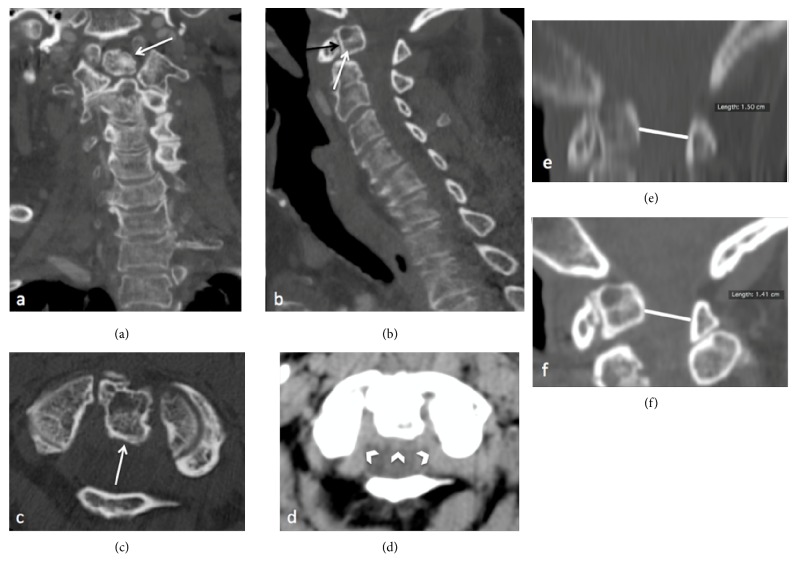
Angiography brain CT obtained to investigate the sudden right hemiparesis of the patient, showing a well-corticated bone fragment located superiorly of odontoid process in coronal reconstruction (a, white arrow) and in axial plane (c, white arrow) in bone window. This fragment seems an os odontoideum, with a pseudoarthrosis of a fracture of the dens as main differential diagnosis. In sagittal plane with bone window, it is associated with an atlantoaxial subluxation at this level (anterior arch of C1 above C2 body), with a narrowing of the space available for spinal cord between the previous 2006 CT exam (e, white line measuring 1.5 cm) and recent 2014 CT exam (f, white line measuring 1.4 cm) in bone window. There is no enlargement of the space between anterior surface of os odontoideum and the anterior arch of C1 on sagittal plane (b, black arrow). We can already see a pseudomass hyperattenuated but without enhancement in the late phase, posteriorly of os odontoideum in the cervical spinal canal in axial plane and soft tissue window (d, white arrowheads), compatible with a retroodontoid pseudotumor in ROP.
